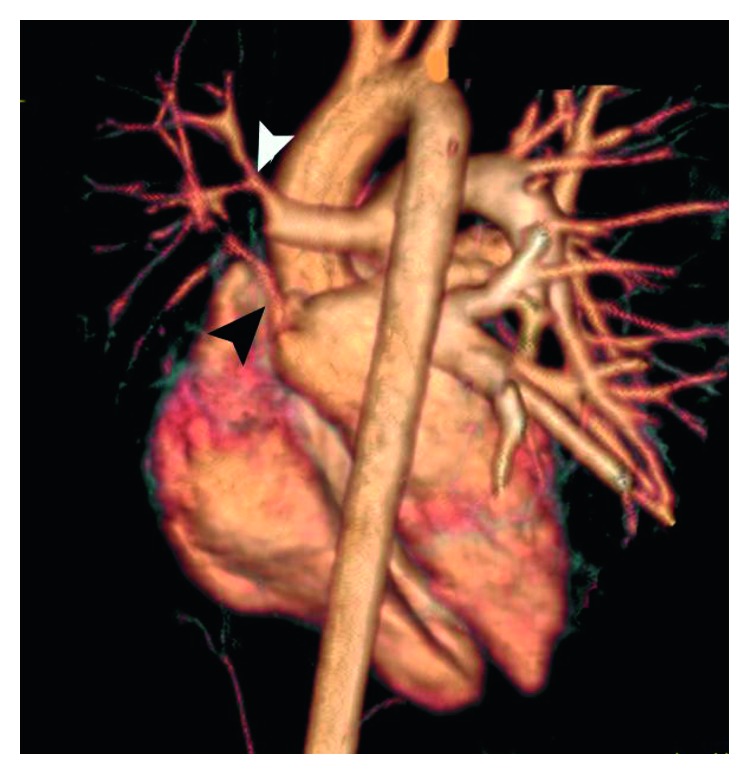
3D reconstruction of the cardiac MRI demonstrates marked narrowing of the right pulmonary artery (black arrowhead) and the right inferior pulmonary veins (white arrowhead).
